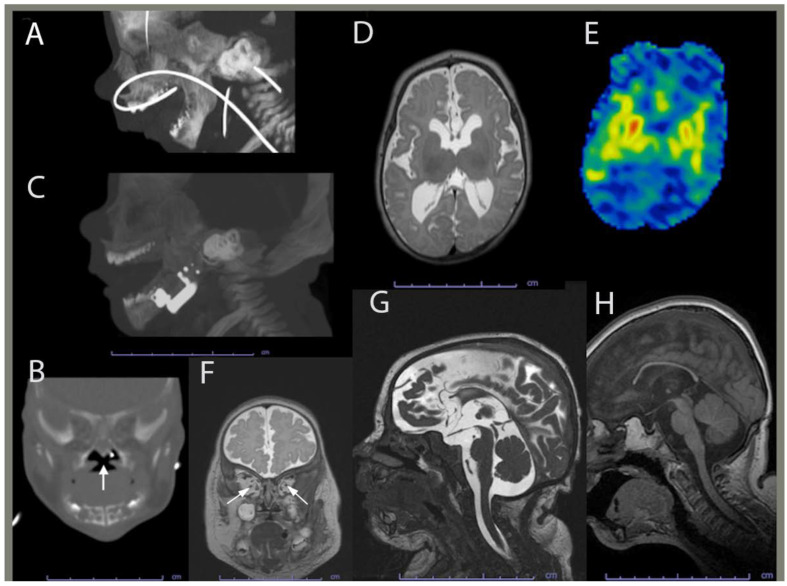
Computed tomography and brain magnetic resonance imaging (MRI) of the proband. (A) Maxillofacial computed tomography (CT) in sagittal planes performed at 35 days shows mandibular hypoplasia. (B) Maxillofacial CT in coronal planes performed at 35 days shows a U-shaped cleft palate with the white arrow indicating components of Pierre-Robin sequence. (C) Sagittal image from a postoperative face CT at 3 months shows improved mandibular and maxillary proportions. (D) Brain MRI axial T2WI image performed at 4 months shows myelination-related signal hypointensity being mildly deficient consistent with hypomyelination or delayed myelination. (E) Brain MRI axial ASL perfusion image showing the basal ganglia being hyperperfused (orange and red hues). (F) Brain MRI coronal T2WI indicating that the optic nerves are thin, consistent with volume loss and/or hypoplasia (arrows). (G) Brain MRI sagittal midline FIESTA image showing moderate diffuse cerebral gray and white matter and mild brainstem volume loss and/or hypoplasia with commissural thinning, decreased white-matter depth, and ex vacuo ventriculomegaly. The corpus callosum is hypogenetic/dysgenetic and hypoplastic, being diffusely thin with absence of the rostrum. (H) Brain MRI at 4 years, showing relatively similar cerebral and brainstem volume loss and/or hypoplasia.
